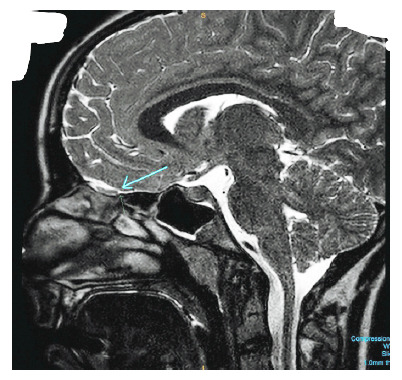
T2-weighted high-resolution image of the MRI brain anosmia protocol sagittal view showing normal volume olfactory bulb with sudden termination and discontinuation, suggesting olfactory tract agenesis (blue arrow).
